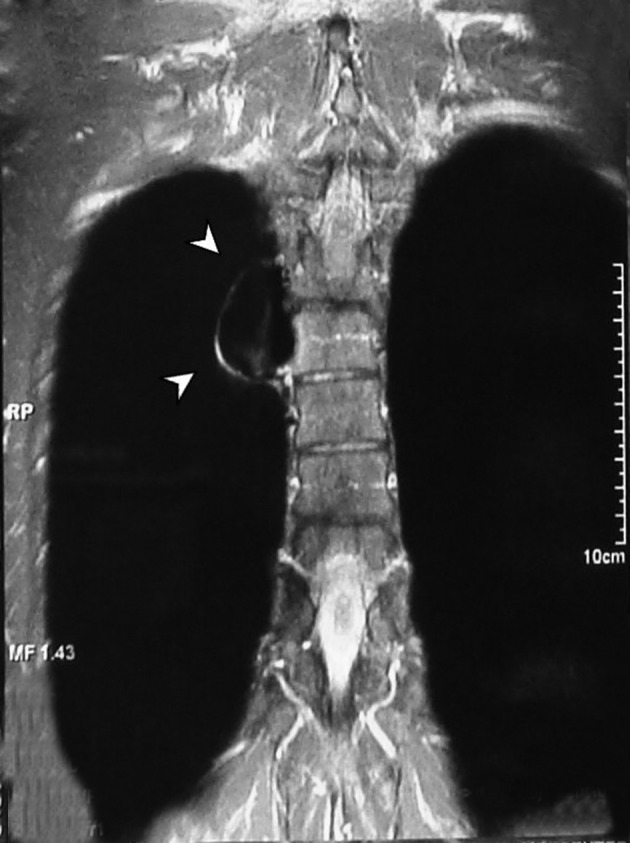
MRI of the thoracic region showing signal void tubular structure in the right paravertebral space
